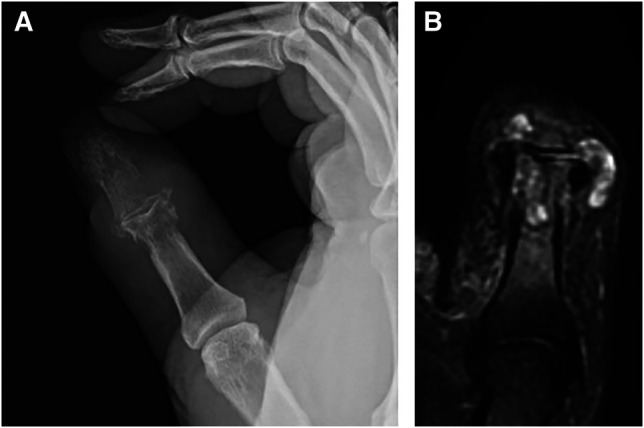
A, Radiographic imaging of gout in the thumb (author's clinical photograph). B, MRI of gout in the thumb (author's clinical photograph).
